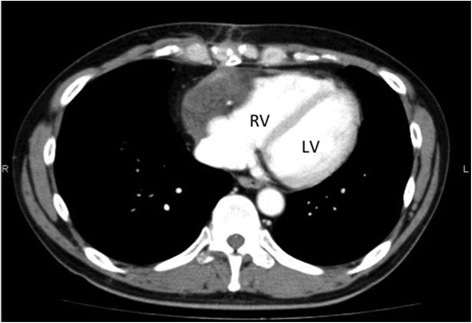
Postoperative chest computed tomography scan, showing volume reduction of the tumor and improvement of the compression of the RV. Abbreviations: *LV* left ventricle, *RV* right ventricle
